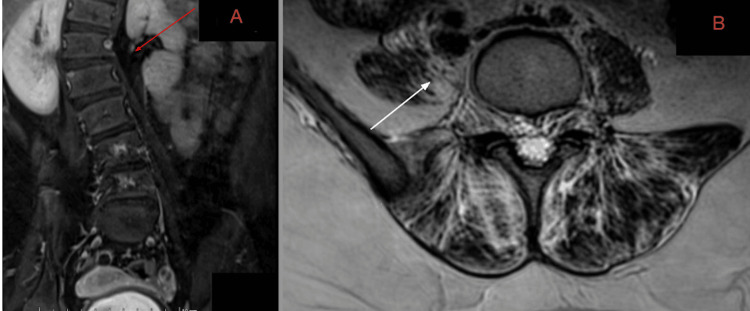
MRI findings in spinal muscular atrophy type III. (A) Coronal MRI demonstrating thoracolumbar spinal curvature (red arrow). (B) Axial MRI demonstrating fatty infiltration and atrophy of paraspinal musculature (white arrow) consistent with neuromuscular disease. MRI: magnetic resonance imaging
